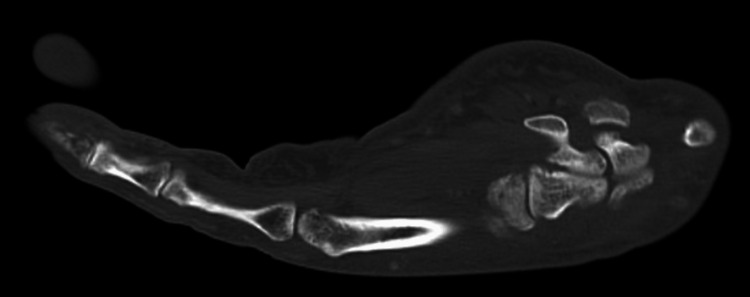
CT left-hand sagittal view with lytic appearance and cortical discontinuity of distal phalanx of index finger concerning for osteomyelitis.
